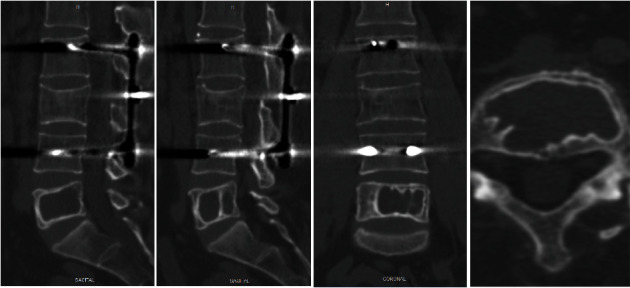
CT sagittal, coronal, and axial images. Sagittal view shows incomplete septum at L5 vertebral body.
